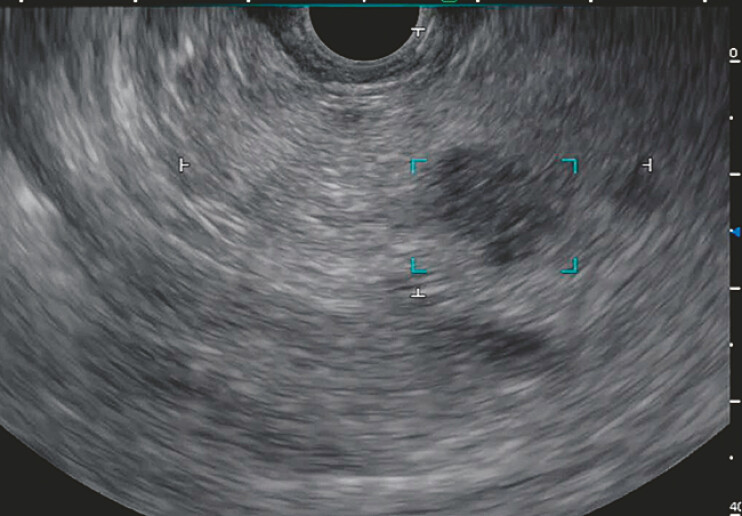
EUS-AI detected the 12 mm lesion in the pancreatic body adjacent to the cyst that had not been detected via MRI. EUS-AI, endoscopic ultrasound system assisted by artificial intelligence; MRI, magnetic resonance imaging.
